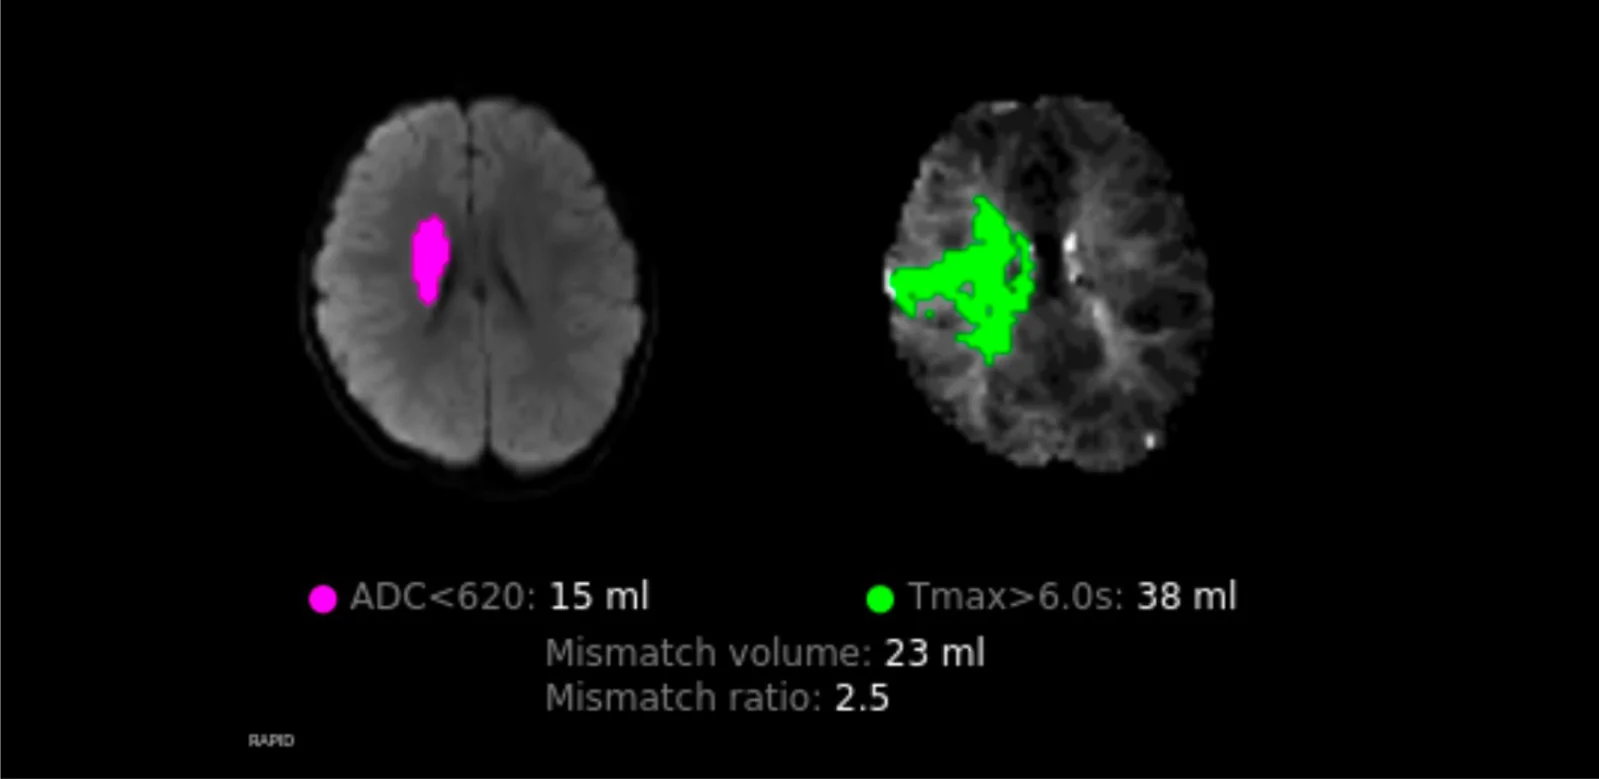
Automated perfusion diffusion software. Example of automated diffusion perfusion MRI in a child with right middle cerebral territory infarction secondary to an M1 segment middle cerebral artery vessel occlusion ADC (left) and perfusion (right) images at three 75 hours post symptom onset showing a 23 mL mismatch between the perfusion and diffusion volumes, representing salvageable brain. ADC, apparent diffusion coefficient; Tmax, time to maximum residue function.
